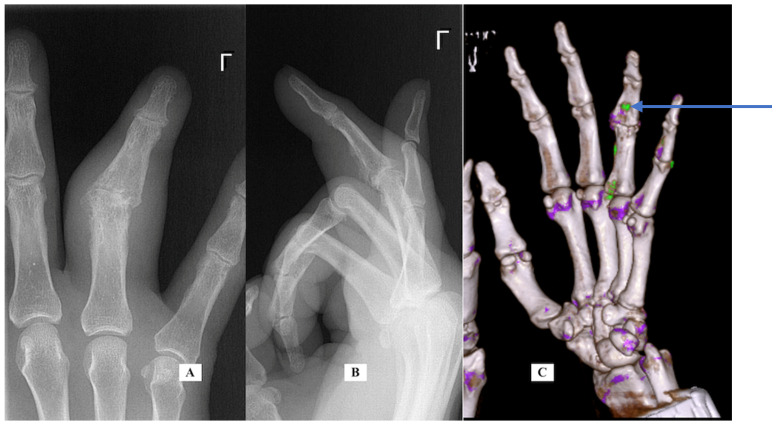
Case 5—X-ray PA (A) and lateral (B) of the finger; CT scan oblique for gout of the hand (C). Only the green color is significant. Purple represents calcifications.
